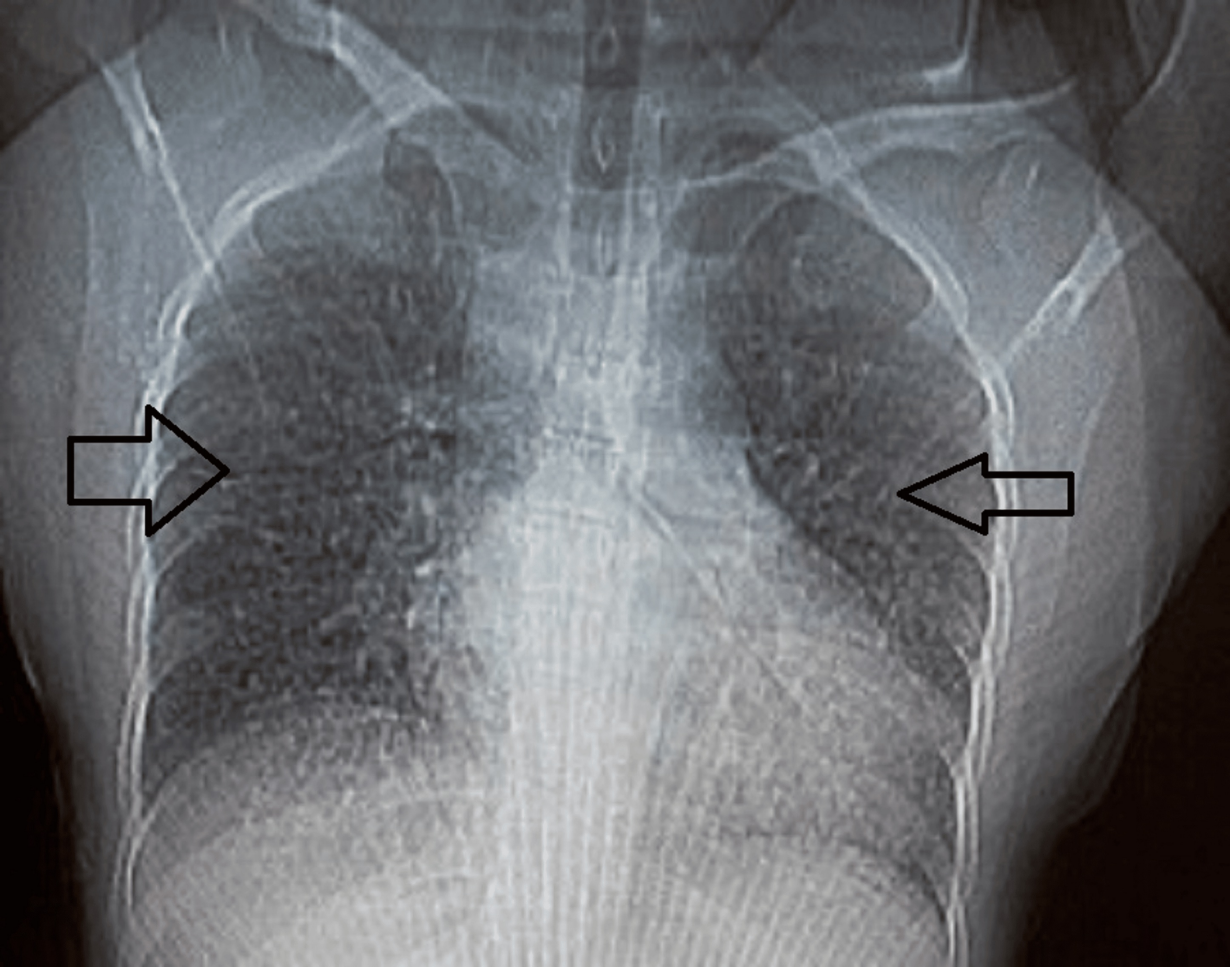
Chest X-ray showing bilateral nodular infiltrate in both lung fields.
